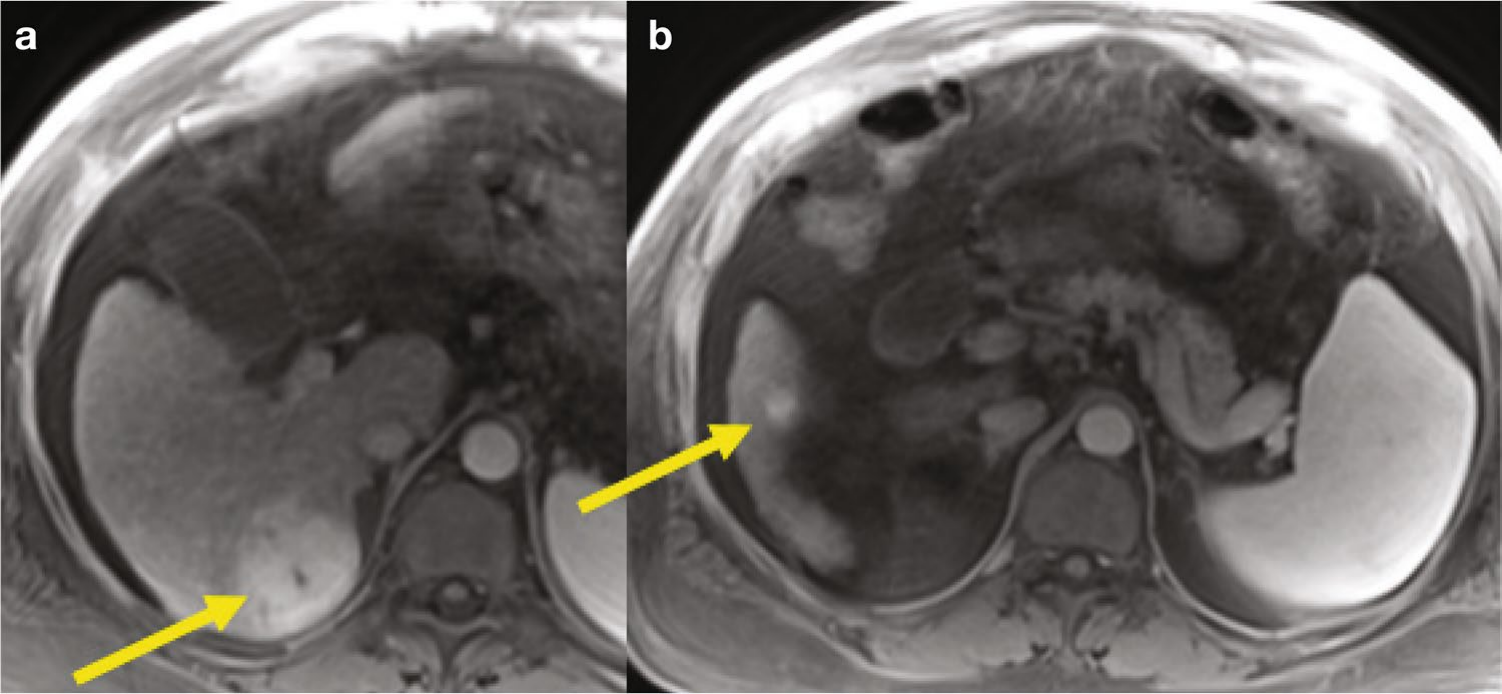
Sixty-year-old male with NASH cirrhosis and HCC. Gadoxetic acid–enhanced T1-weighted images during the arterial phase at two different levels demonstrate two arterially hyperenhancing lesions in the right hepatic lobe (a: segment 7 and b: segment 6, arrows), compatible with HCC (later phases not shown). There is background cirrhosis and perihepatic ascites. *Abbreviation: HCC,* hepatocellular carcinoma
